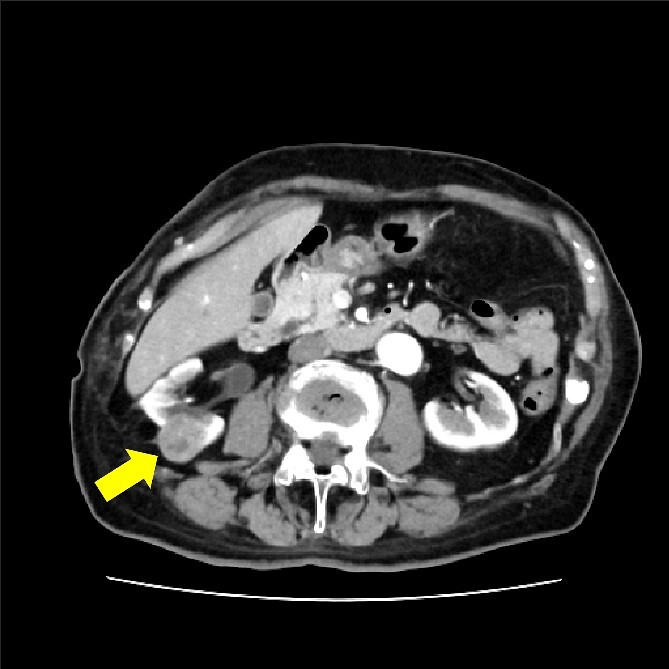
CT showing 30 mm right renal cell carcinoma. The arrow shows the tumor.
